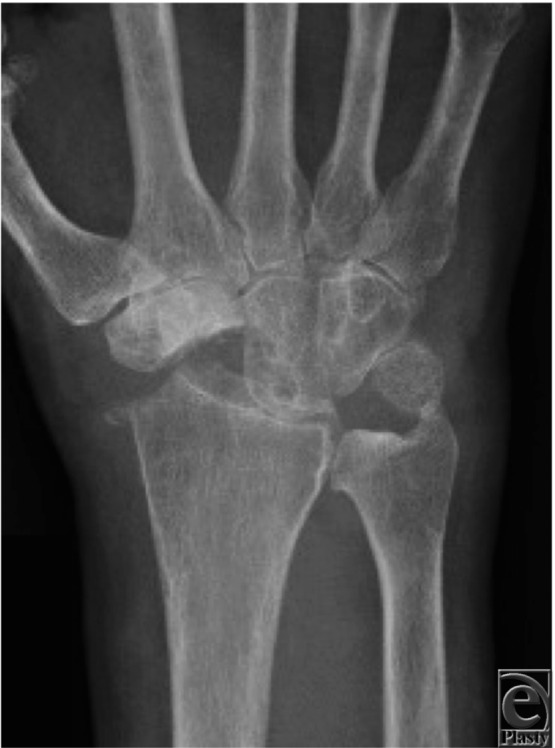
Postoperative posteroanterior radiograph showing satisfactory positioning of the capitate in the lunate facet.
